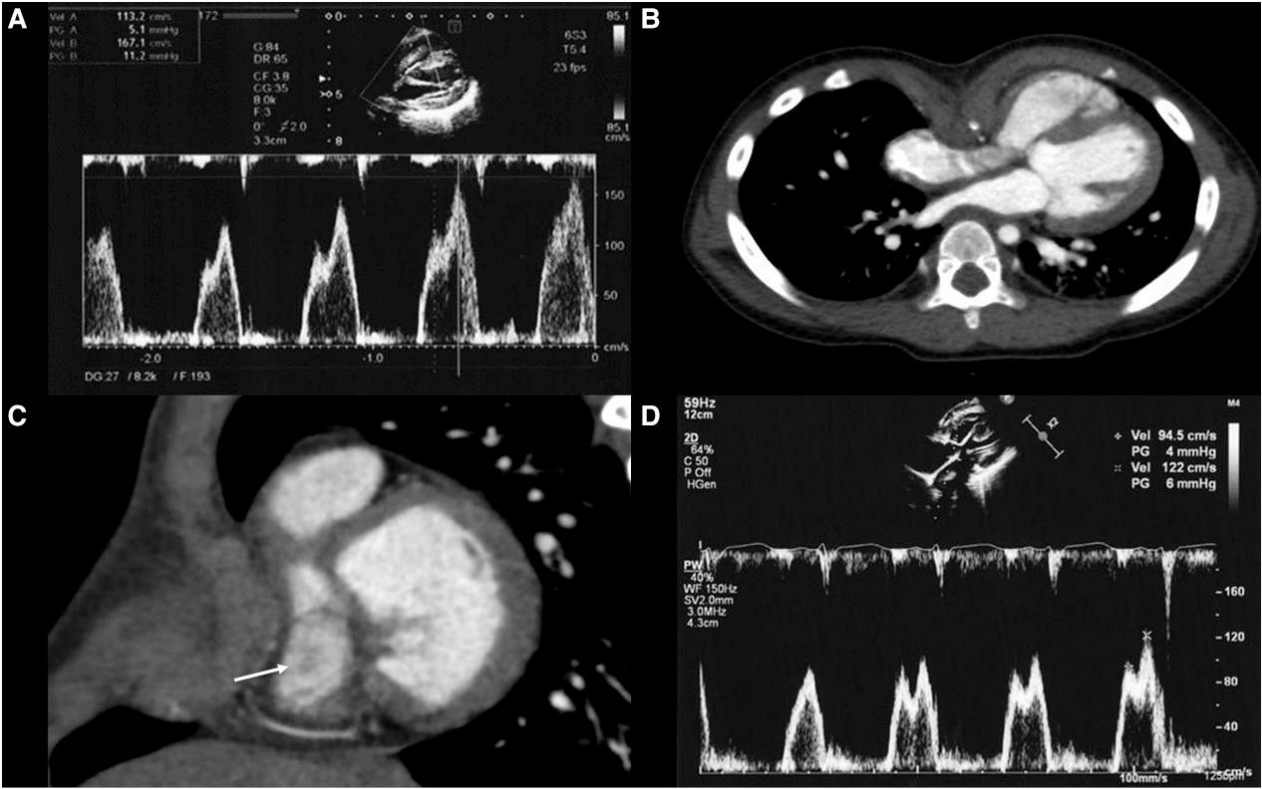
(*A*) Pulsed Doppler image of tricuspid valve inflow blood flow before pectus excavatum surgery. (*B*) Horizontal sectional image of chest contrast-enhanced computed tomography before pectus excavatum surgery. The Haller index was 6.1. (*C*) Chest contrast-enhanced computed tomography image of the cross-sectional view looking down at the tricuspid valve (arrow) from the right atrium side before pectus excavatum surgery. (*D*) Pulsed Doppler image of tricuspid valve inflow blood flow after pectus excavatum surgery.
